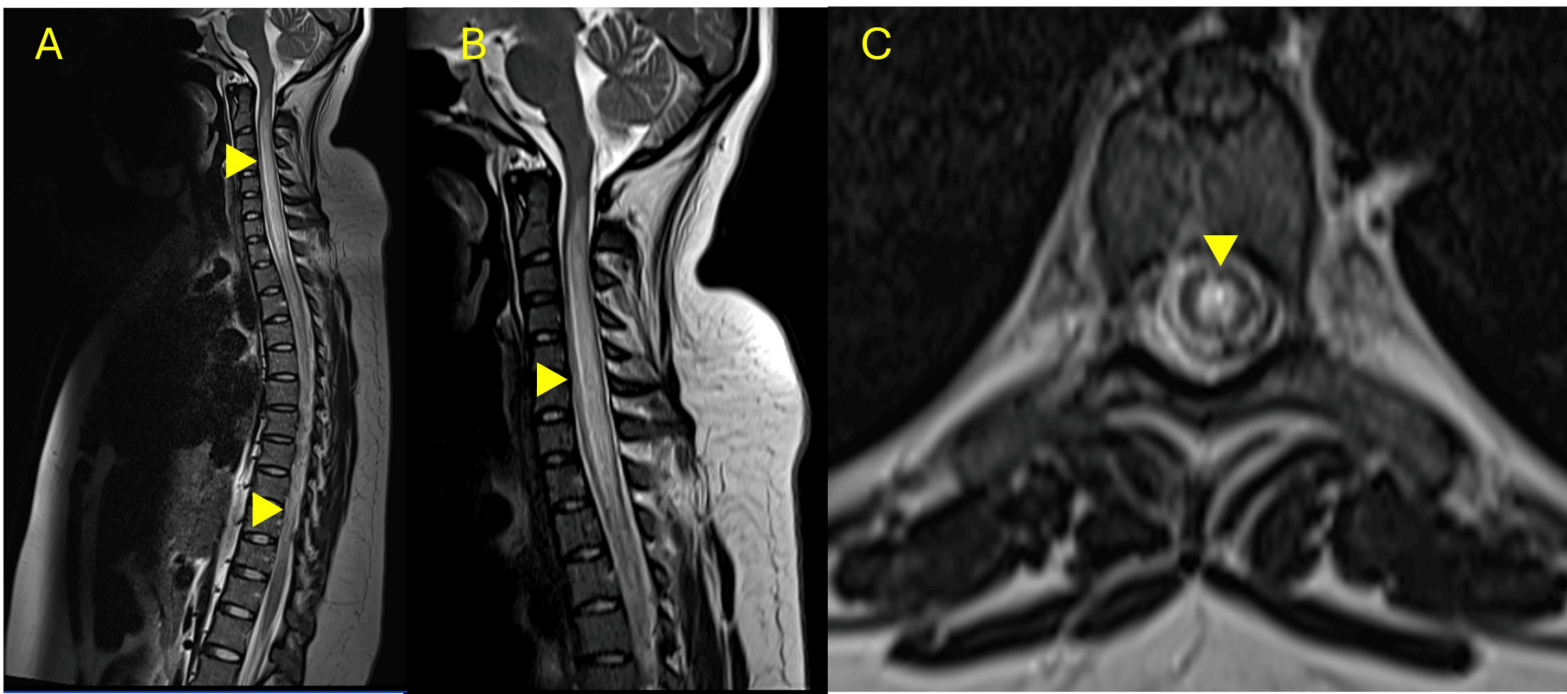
Spinal MRI findings at initial presentation showing LETM. A. Sagittal T2-weighted MRI of the cervicothoracic spine shows increased signal intensity and swelling of the spinal cord extending from C1–C2 to T11–T12 (yellow arrowheads), consistent with LETM. B. Sagittal T1-weighted MRI with gadolinium contrast demonstrates mild, homogeneous enhancement of the cervical lesion (yellow arrowhead), without evidence of mass effect or hemorrhage. C. Axial T2-weighted image at the thoracic level reveals central hyperintensity within the spinal cord (yellow arrowhead), indicating intramedullary involvement. LETM, longitudinally extensive transverse myelitis
